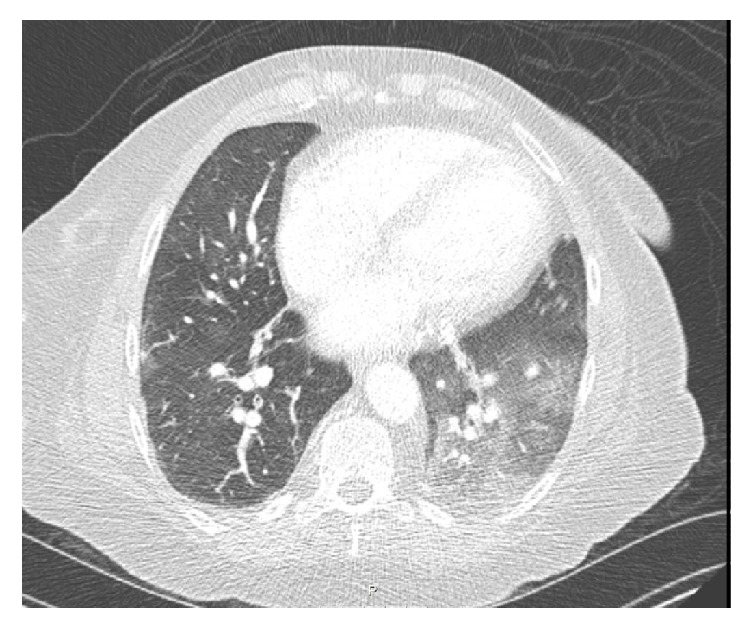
CT chest showing ground glass opacities predominantly on the left side (case 1).
